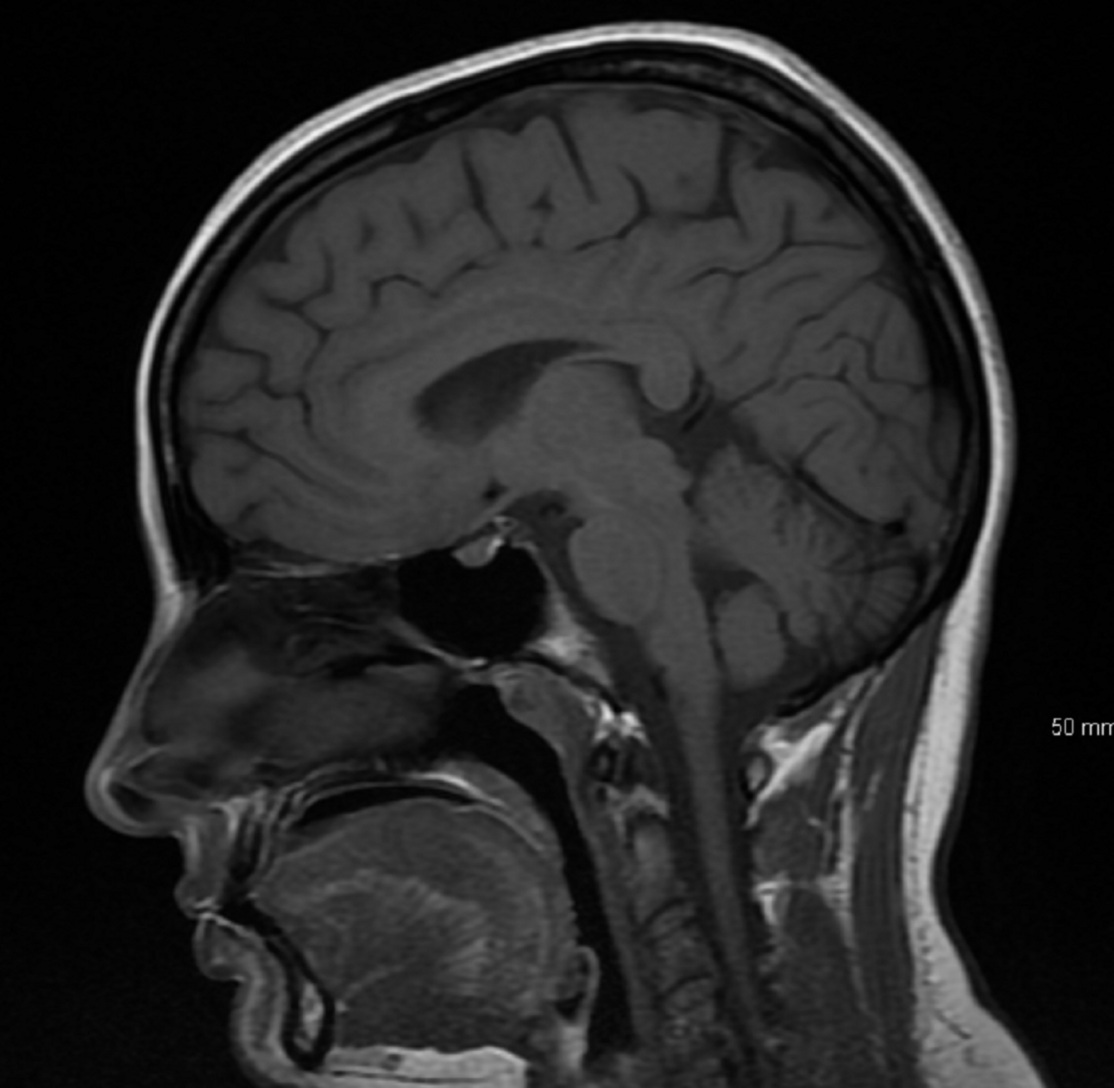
MRI brain sagittal section showing normal brain with no enhancement (Coronal section however showed hyperintense lesion as in Figure 1).
